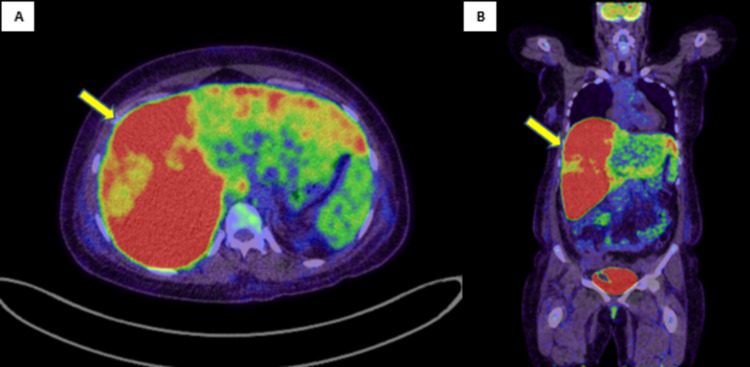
(A) Axial and (B) coronal PET CT showing intense diffuse uptake of FDG in the right lobe suggestive of diffuse involvement (yellow arrows).
